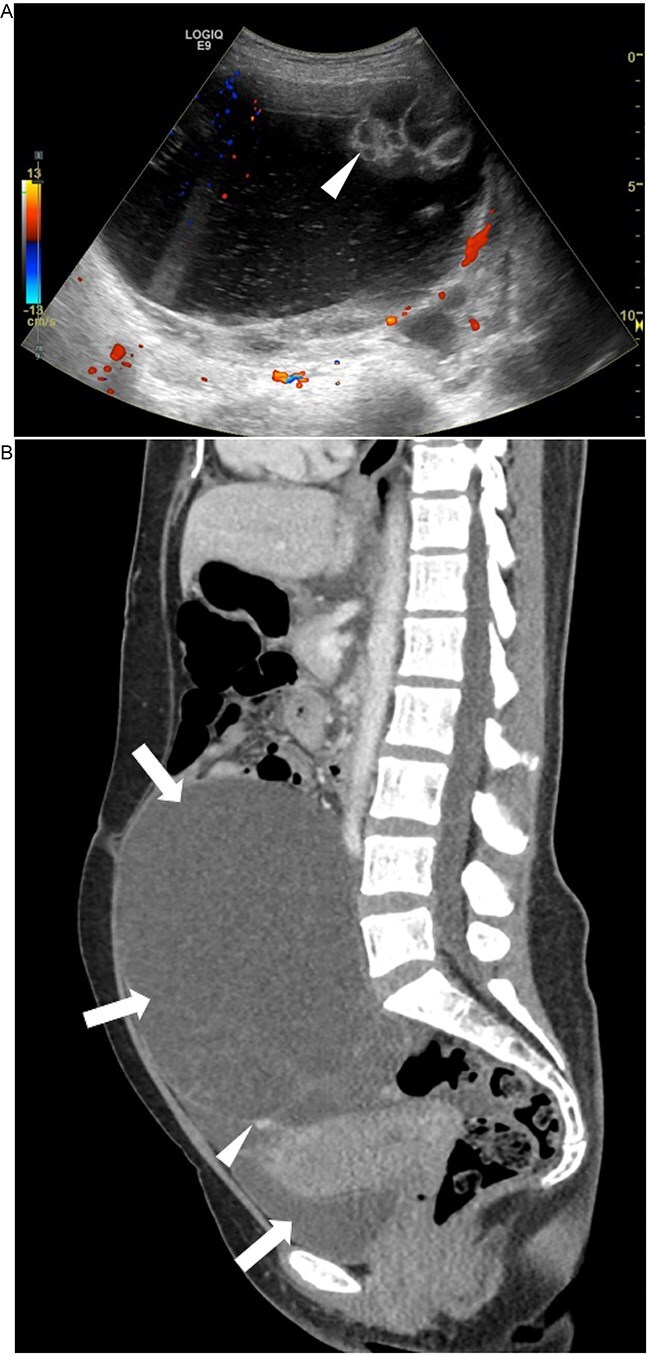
CT: Sagittal CT image demonstrates an approximately 19 cm cystic structure (arrows) occupying the lower abdomen and pelvis. There is suggestion of either complex septations and/or solid material (arrowhead).
